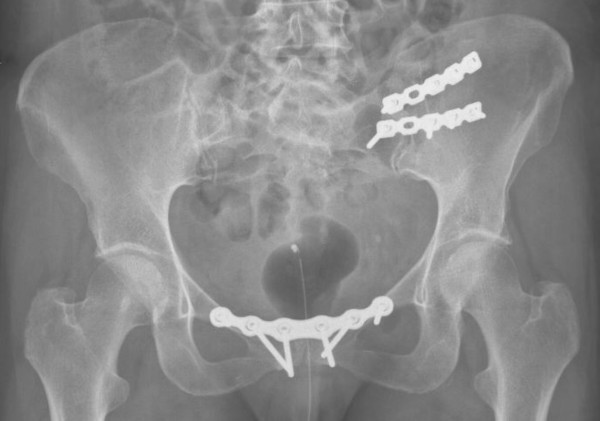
Post-operative X-ray of SIJ fusion with concomitant fusion of the pubic symphysis.
